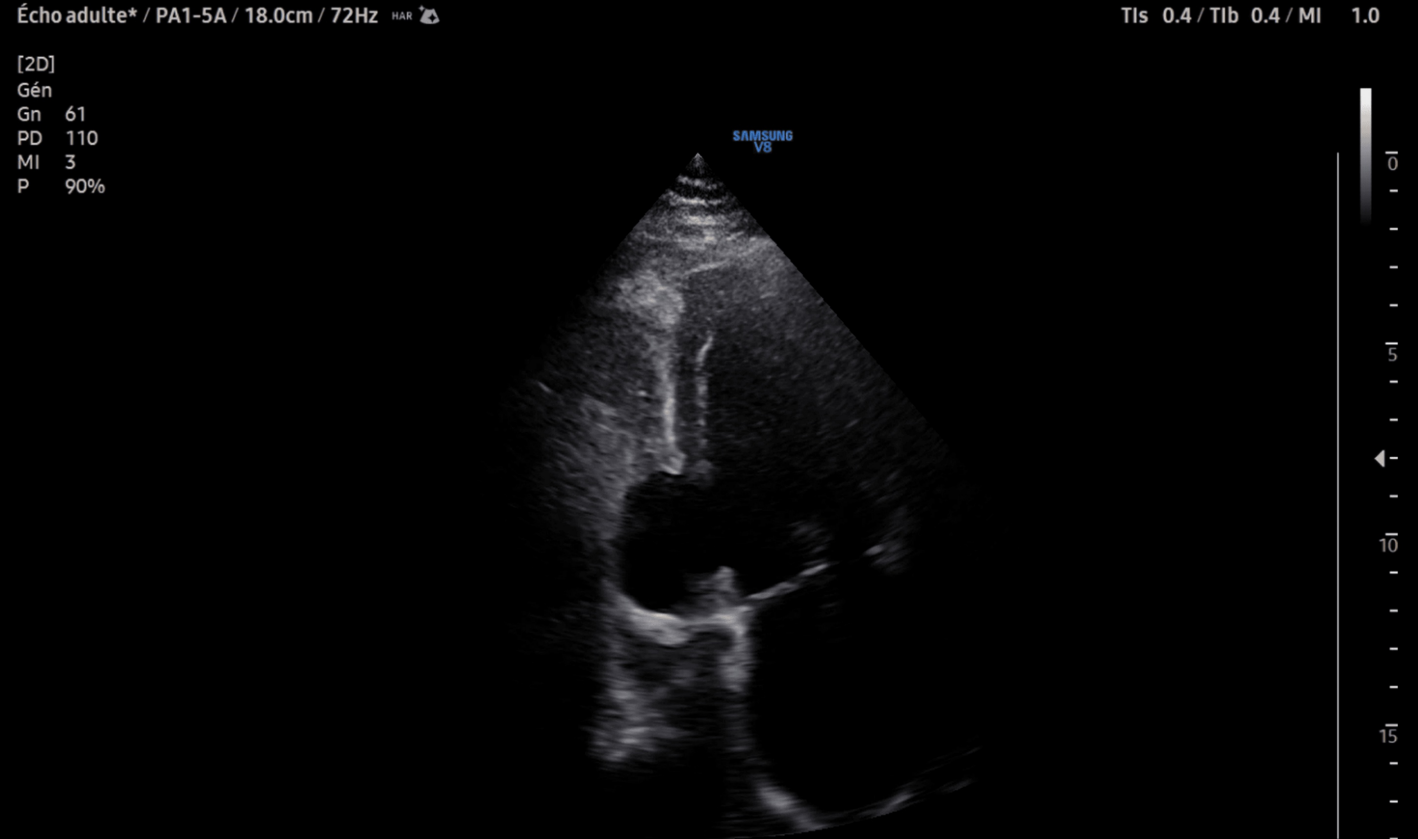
Two-chamber transthoracic echocardiographic view showing a left ventricular pseudoaneurysm consistent with contained myocardial rupture
